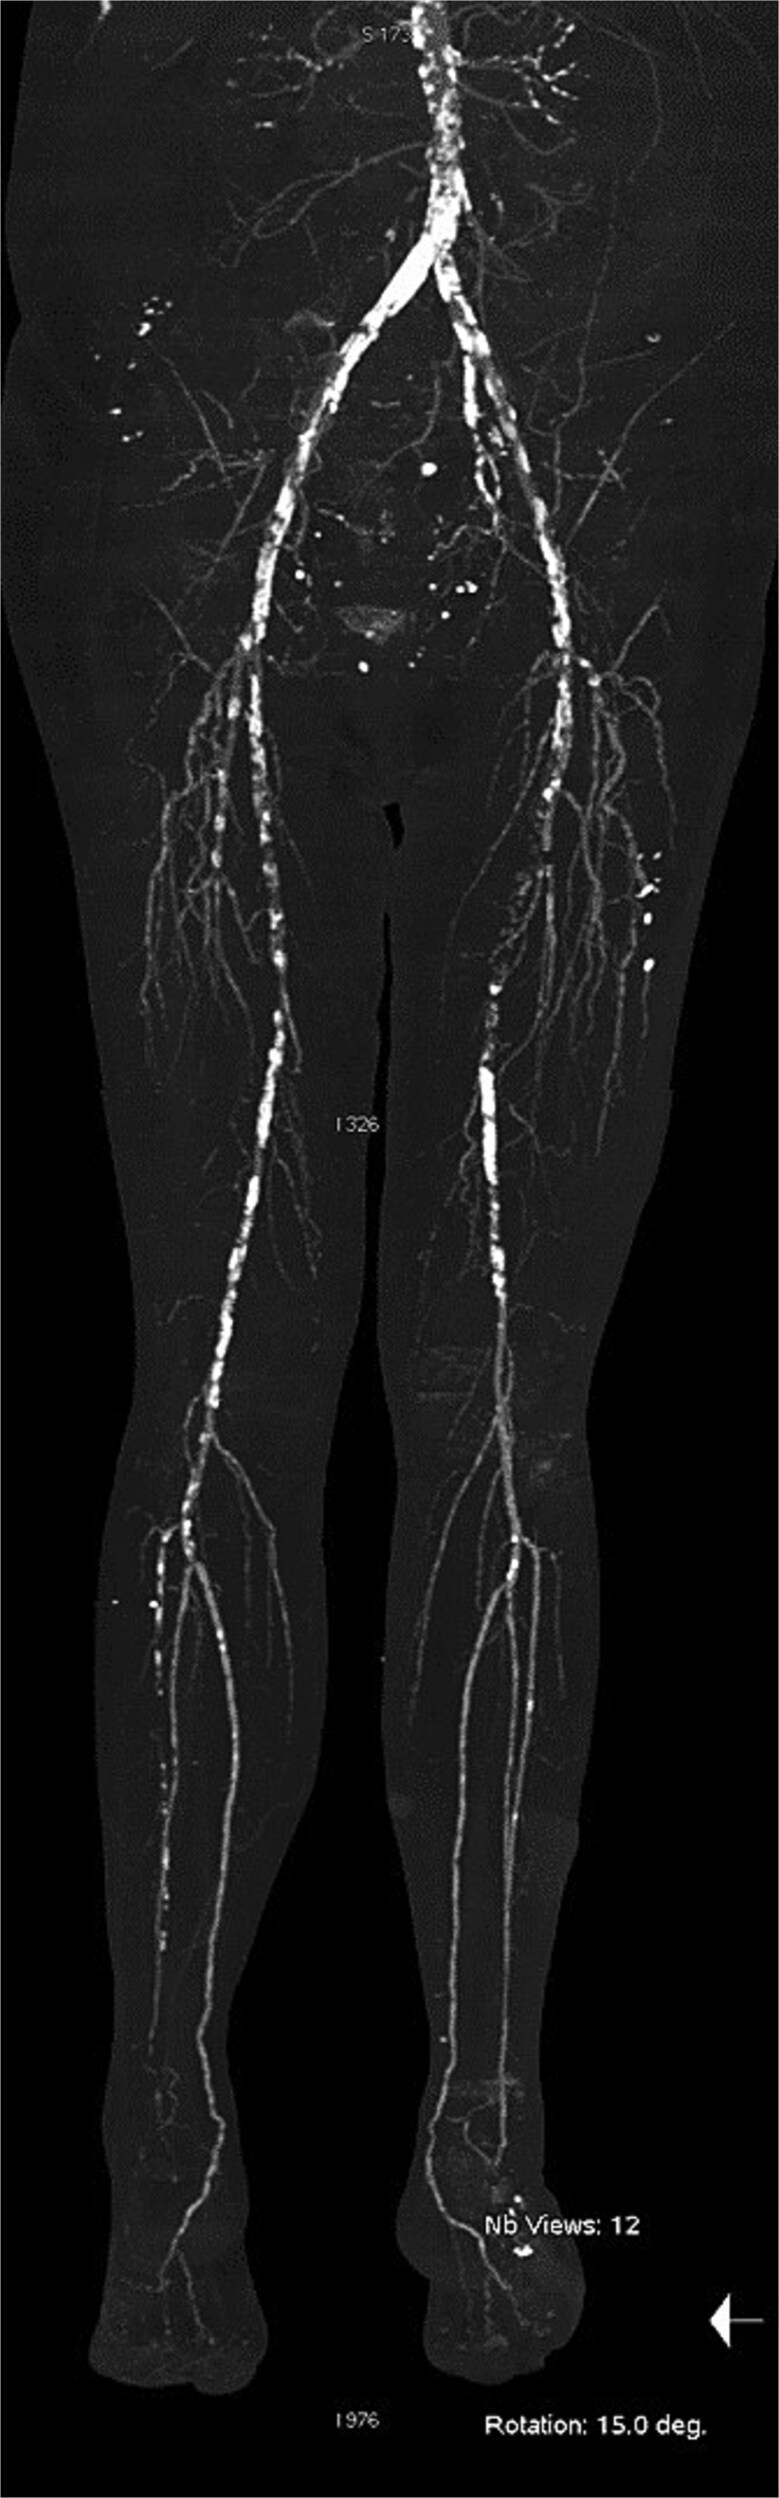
Computed tomography angiography at the time of readmission.
